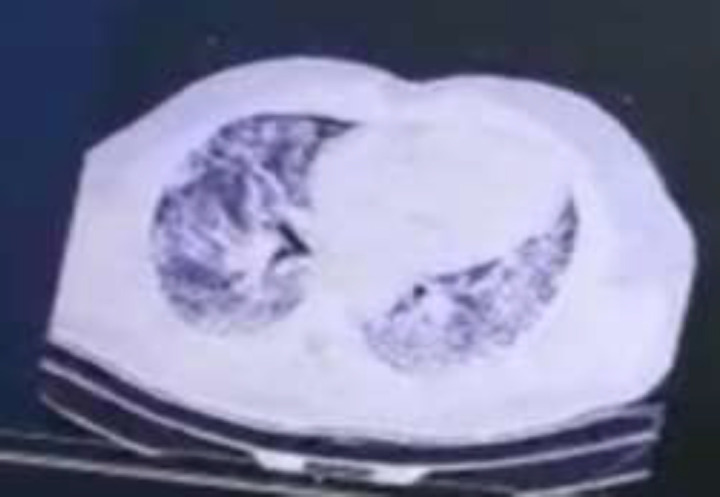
Chest CT scan of a 74-year-old male showed bilateral GGOs
